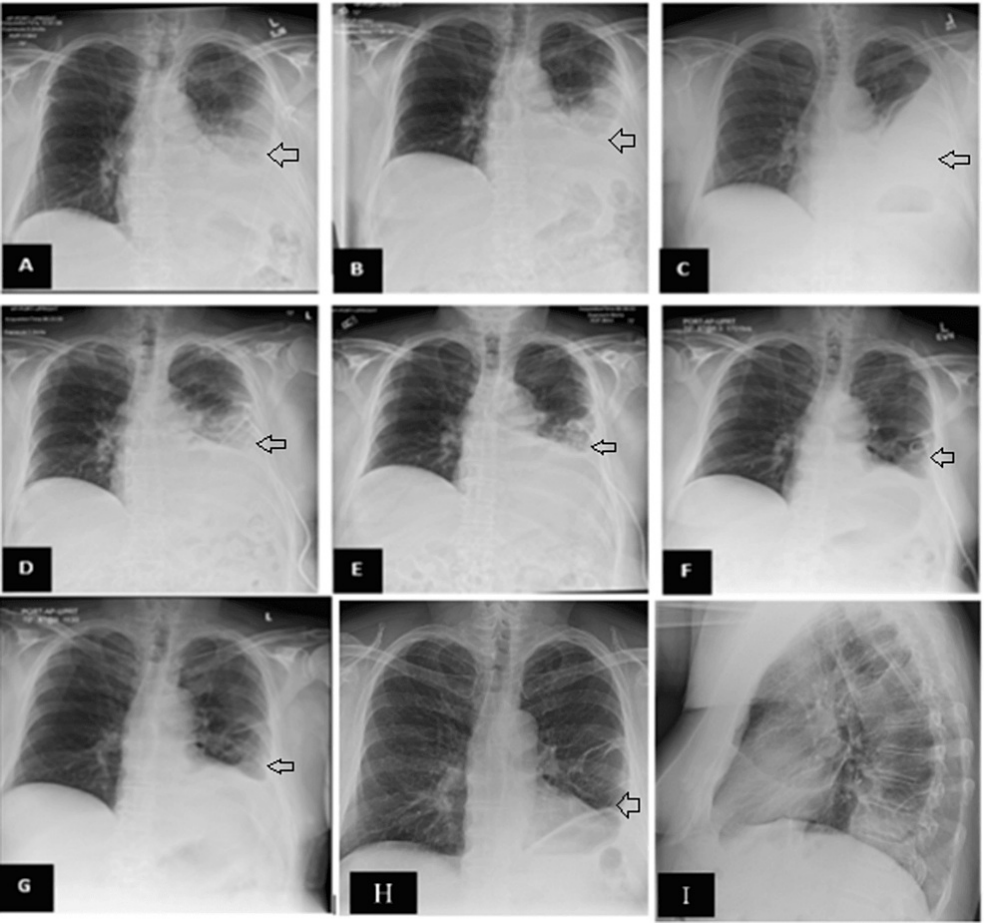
Serial Chest X-rays (A) Day two. (B) Day two post thoracentesis. (C) Day four. (D) Day six following placement of chest tube. (E) Day nine following completion of fibrinolytic therapy. (F) Day 15 following placement of a larger caliber chest tube. (G) Day 17 after removal of a chest tube. (H) Day 26 post-discharge showing a small residual effusion. (I) Day 26 post-discharge lateral chest x-ray. Arrows point to the patient's pleural effusion.
